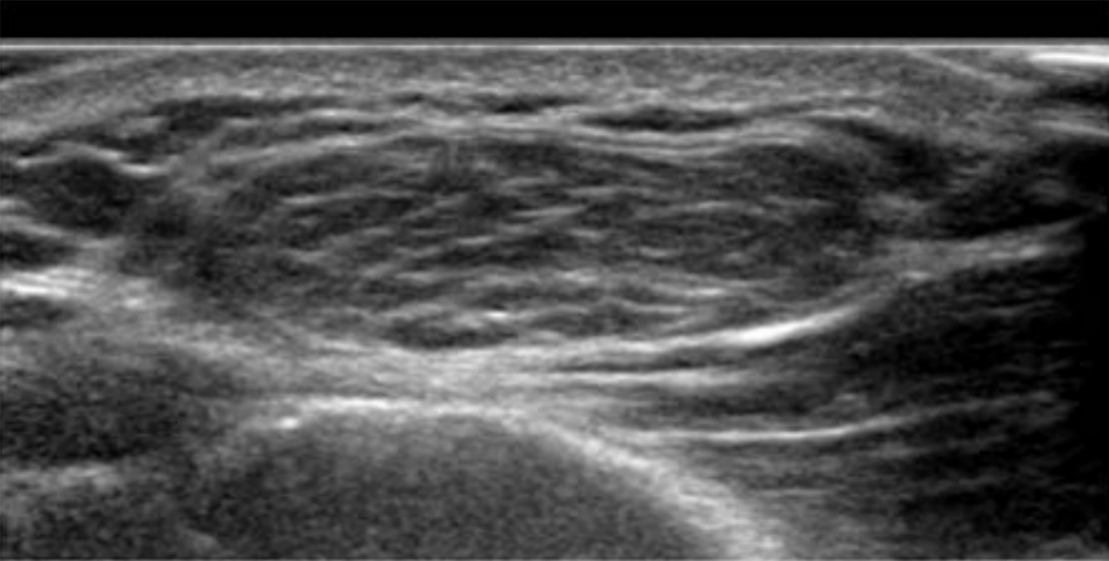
Example of benign lipoma with sonographic appearance of well organized, heterogeneous echogenicity with multiple clearly defined long smooth continuous internal echogenic lines parallel to the long axis of the lesion superficial to any musculature 20.
